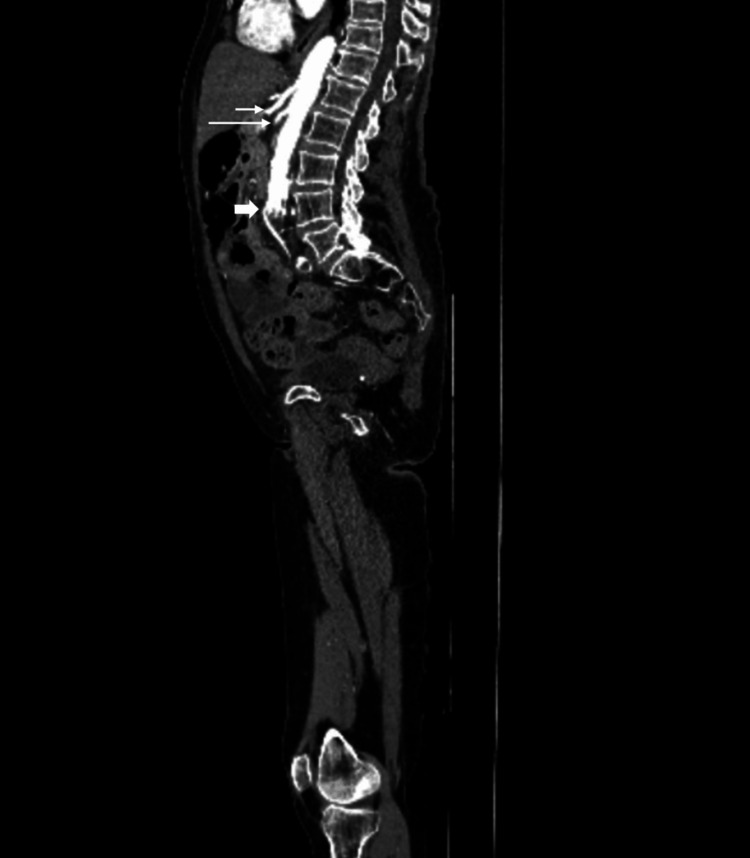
A sagittal section of computed tomography angiography (CTA) showing a patent origin of the coeliac trunk (short white arrow), superior mesenteric artery (long white arrow), and inferior mesenteric artery (thick white arrow).
